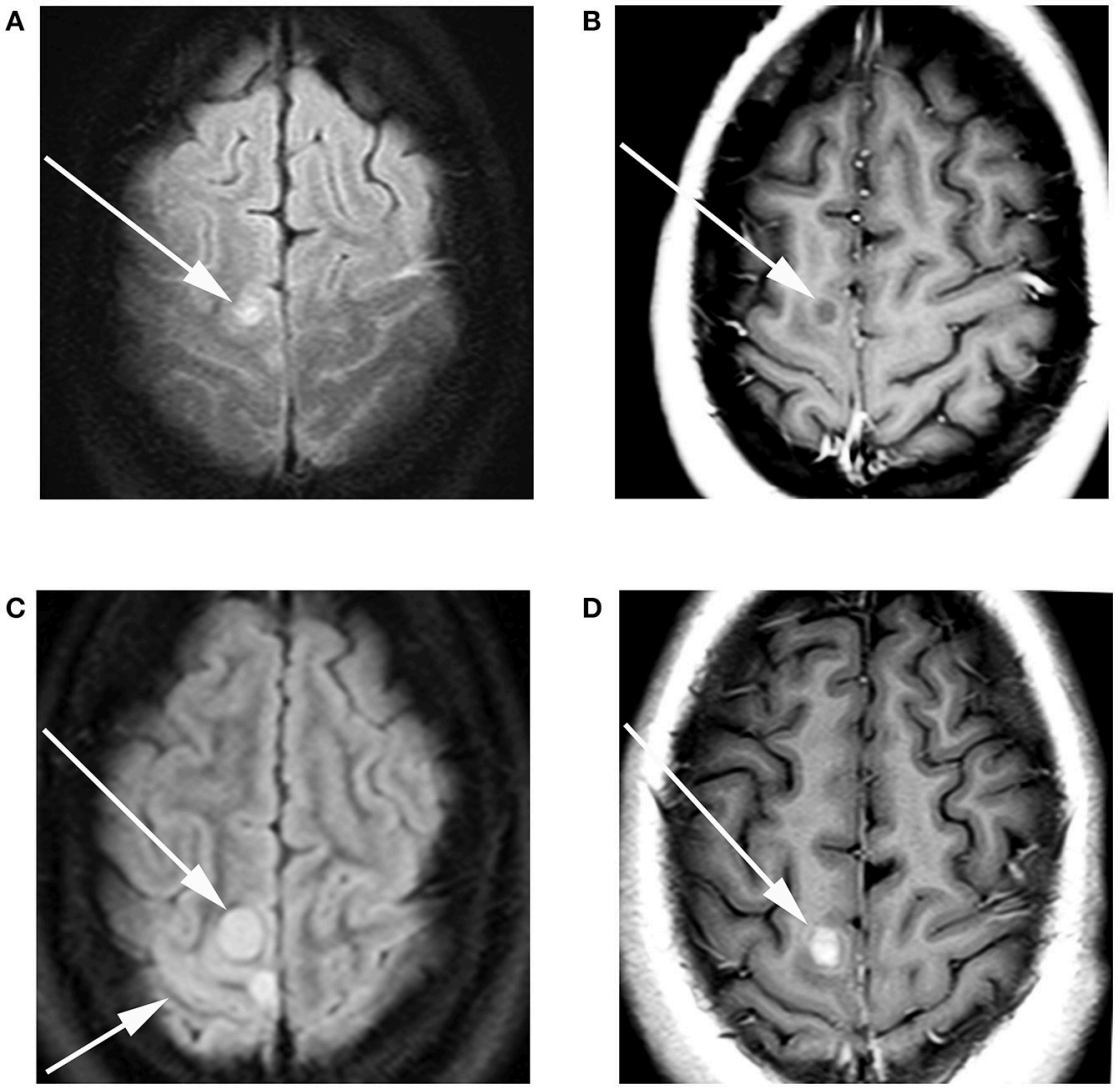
A 17-year-old female with H3F3A G34R mutated infiltrative glioma. MRI of the brain with and without contrast at the time of presentation revealed (A) a T2 FLAIR hyperintense focus (arrow) with adjacent T2 FLAIR hyperintense cortical thickening. (B) T1 contrast-enhanced imaging revealed faint peripheral enhancement (arrow). Three-month follow-up imaging revealed (C) increase in the size of the T2 FLAIR hyperintense lesion long arrow with associated cortical thickening short arrow. (D) T1 contrast-enhanced imaging revealed a solid enhancing nodule (arrow).
